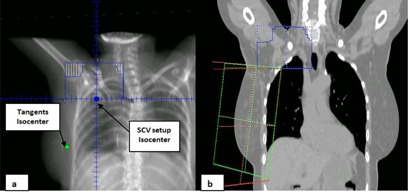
Three‐field setup using two‐isocenter technique. (a) The digitally reconstructed radiographs (DRR) of the coronal beam's eye view (BEV) showing the positions of the tangents and SCV isocenters. The MLC was used to shield the trachea and shoulder joint. (b) The coronal view of the planning CT showing the matching between the SCV field (blue) and tangent field borders (red and green).
